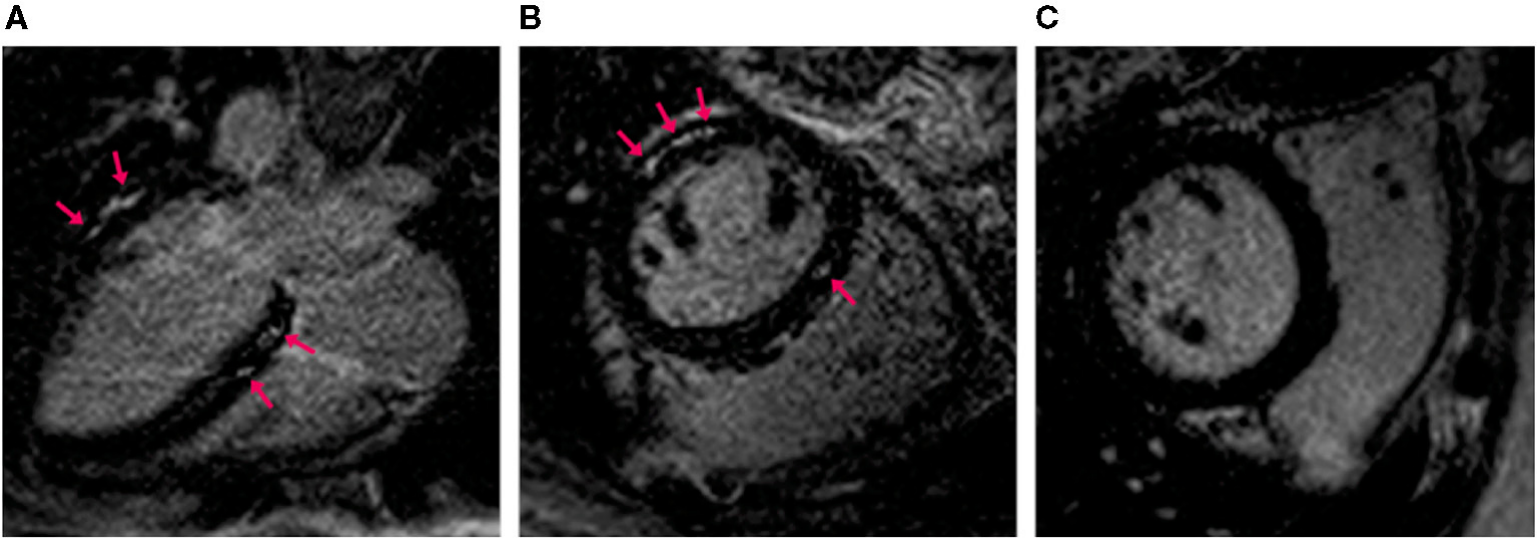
Magnetic resonance images of the heart with late gadolinium enhancement. Long axis (A) and short axis (B,C) images. (A,B) show myocardial fibrosis (arrows) in a 46-year-old woman. (C) shows the corresponding findings in a 42-year-old unaffected woman.
